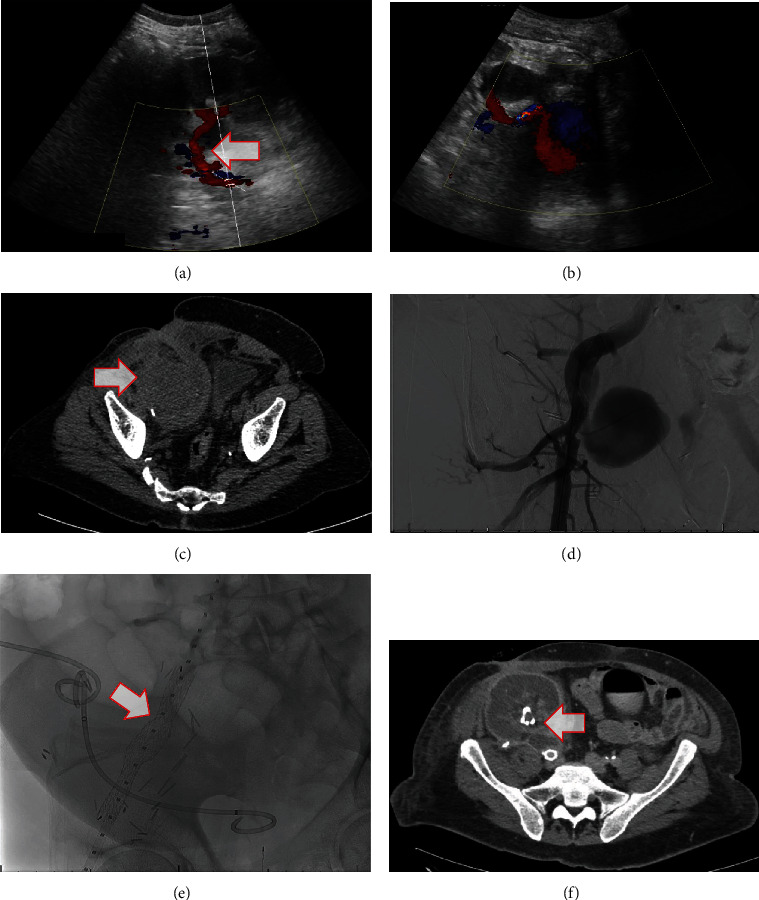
Case 2 imaging, whose blood and urine cultures on admission grew *Enterococcus faecalis*. (a) Ultrasound from initial transplant hospitalization demonstrating normal RLQ transplant kidney with patent renal artery and vein without pseudoaneurysm. (b) Ultrasound on admission demonstrating pseudoaneurysm at the anastomosis measuring 5 cm × 4 cm × 6 cm. (c) Noncontrast CT demonstrating 8 cm × 8 cm hematoma in the same area of the pseudoaneurysm seen on ultrasound approximately 4 hours earlier. (d) Arteriogram demonstrating 5 cm × 5 cm juxta-anastomotic pseudoaneurysm; it was deemed unsafe to coil given its wide neck. (e) Arteriogram on the following day after placement of a 16 mm × 14.5 mm × 10 cm Gore excluder endograft. (f) CT angiogram demonstrating endograft placement completely excluding the anastomotic pseudoaneurysm.
